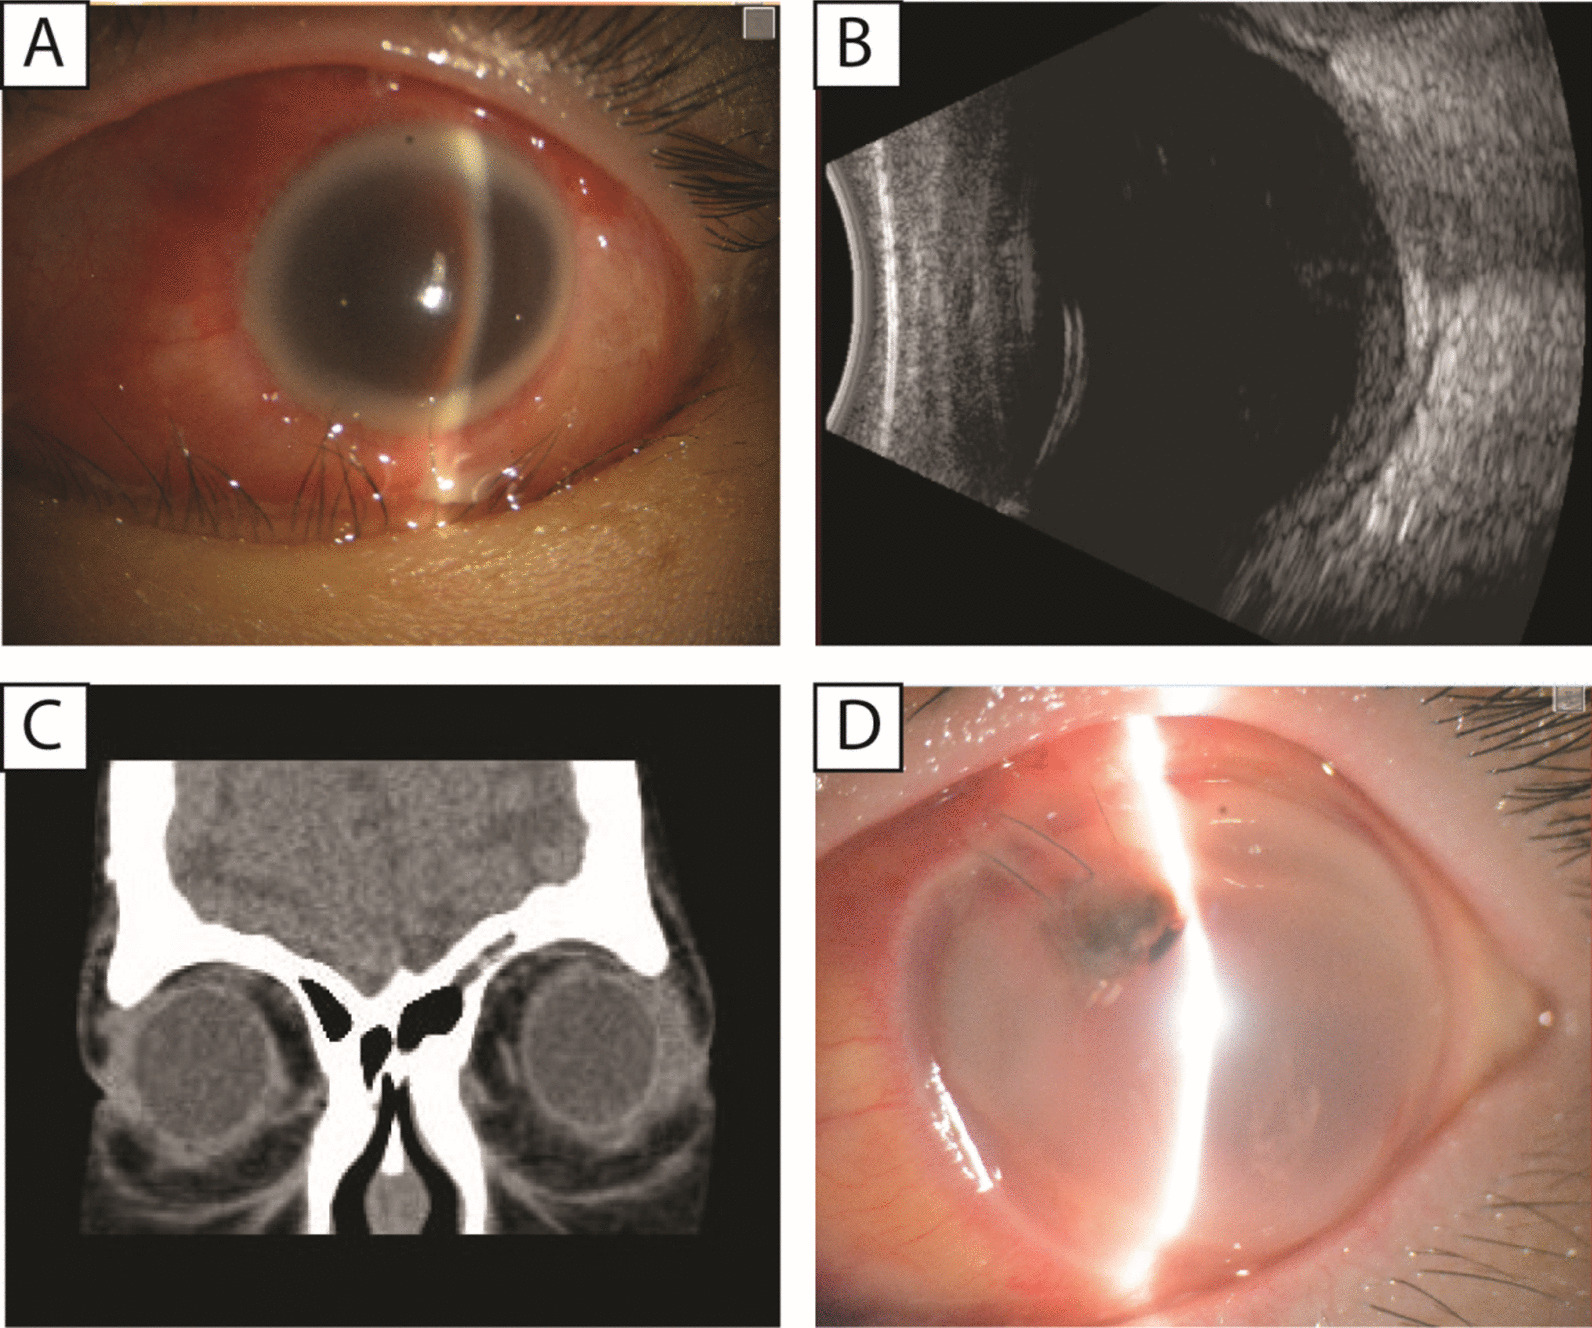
A Slit-lamp examination revealed severe conjunctival hyperemia, corneal edema, and hypopyon. B Ultrasound B scans showed dotted, clustered low to moderate echogenicity in the posterior vitreous and edema and thickening of the bulbous wall. C Computed tomography scan showed periocular tissue swelling. D Severe corneal ulceration and perforation
